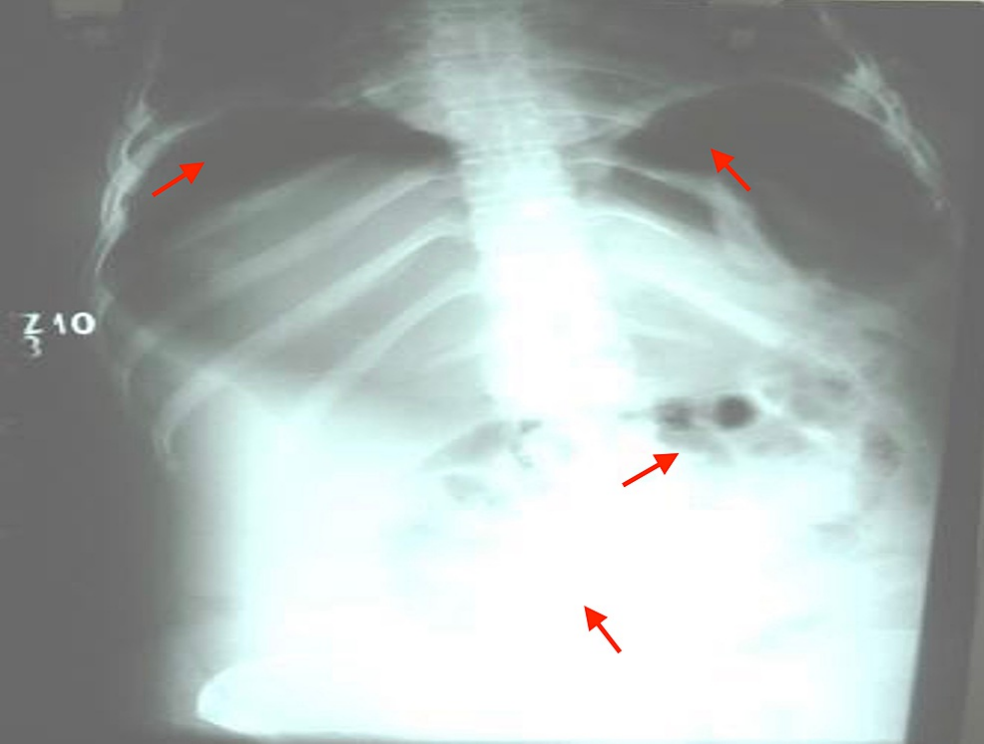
Chest and abdomen (erect) radiograph reveals air under diaphragms, diffuse opacity in the abdomen, and a few gas-filled bowel loops in the abdomen (red arrows)
